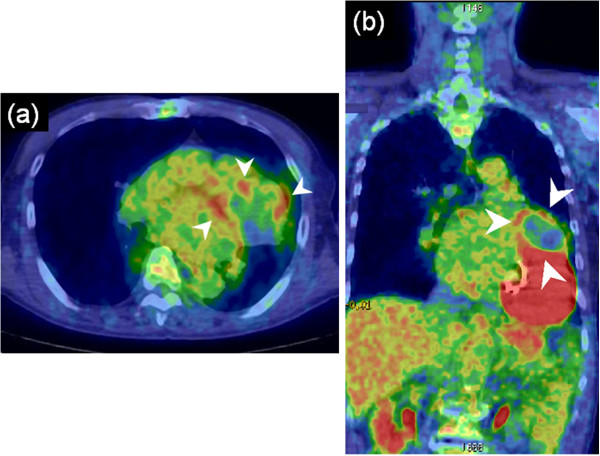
Fused positron-emission tomography (PET) and computed tomography (CT) image. (a) Axial fused PET and CT image shows 2-[^18^ F] fluoro-2-deoxy-d-glucose (FDG) uptake in the peripheral rim of the mass (arrowheads). The maximum standardized uptake value (SUV) of this lesion was 3.50. (b) Coronal fused PET and CT image. Other signs of abnormal uptake suggesting a malignant lesion were not observed in other intrapericardial mass (arrowheads).
